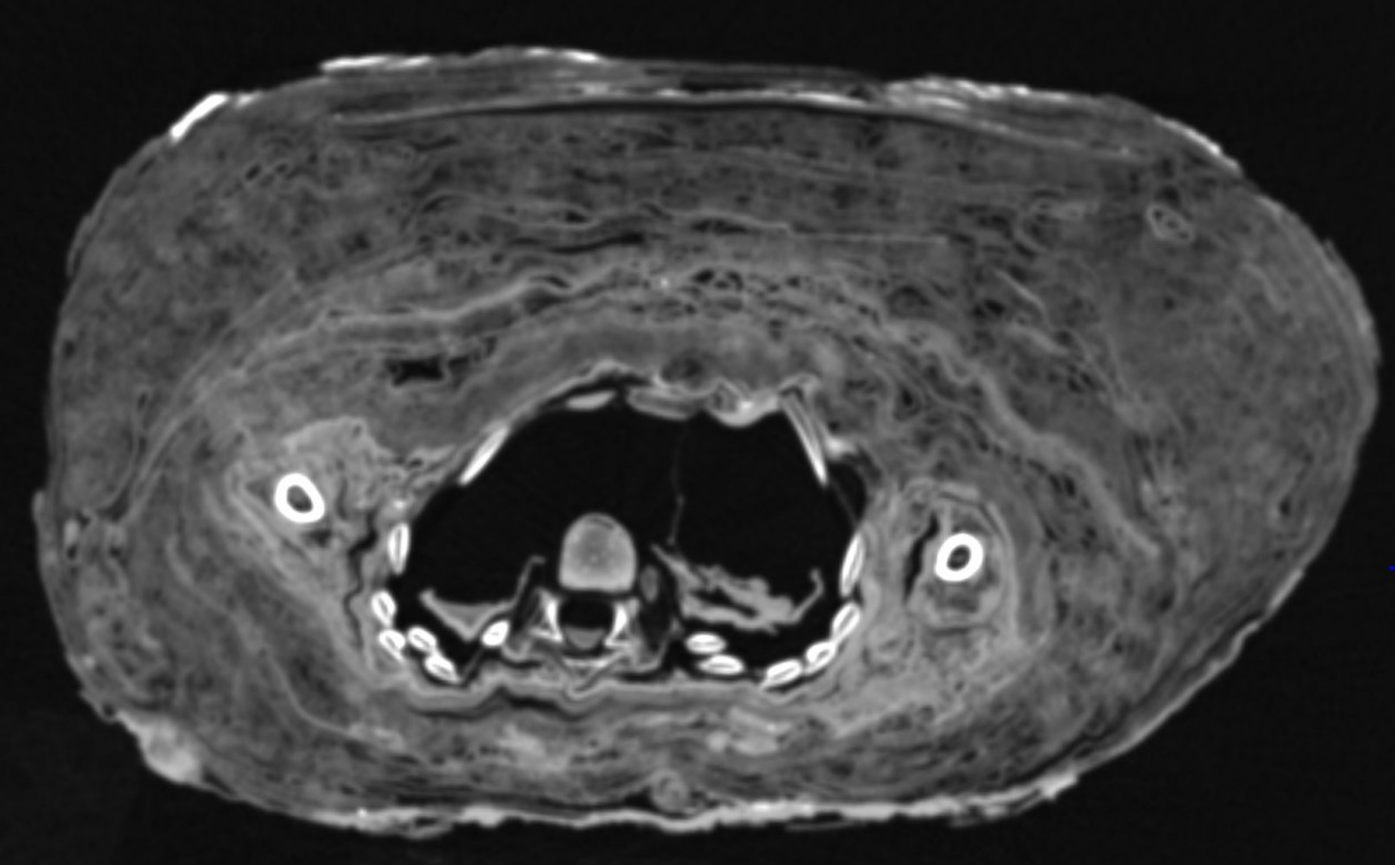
CT-scan through the chest. This section shows the residues of both lungs; the right lung being patchy condensed.
